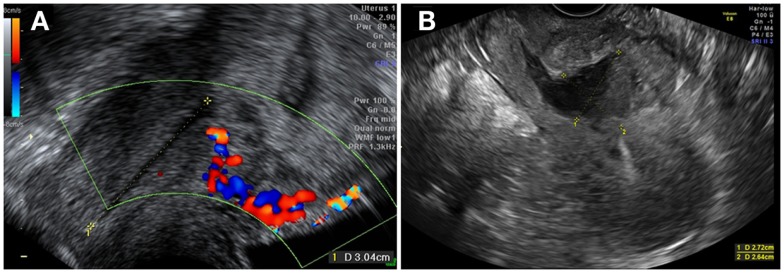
(A) Intra-uterine pregnancy at 7 weeks of gestation, with trophoblastic insertion in the cesarean scar site, with a Doppler flow underneath. (B) Distended uterine scar after D&C.
